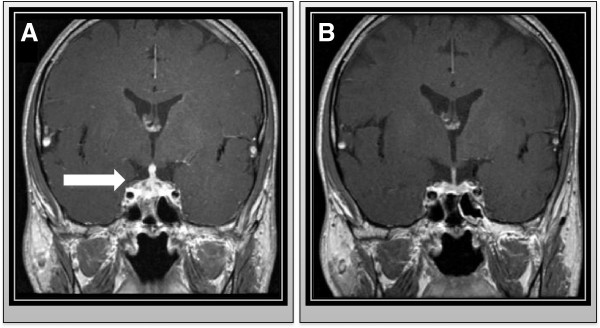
NMR scan of enlarged pituitary at diagnosis and after treatment. A. Contrast-enhanced coronal T1-weighted nuclear magnetic resonance (NMR) scan of the sella turcica showing pituitary and pituitary stalk enlargement with inhomogeneous contrast enhancement. B. Contrast-enhanced coronal T1-weighted nuclear magnetic resonance (NMR) scan of the sella turcica two months after hypophysitis demonstrates normal pituitary size.
